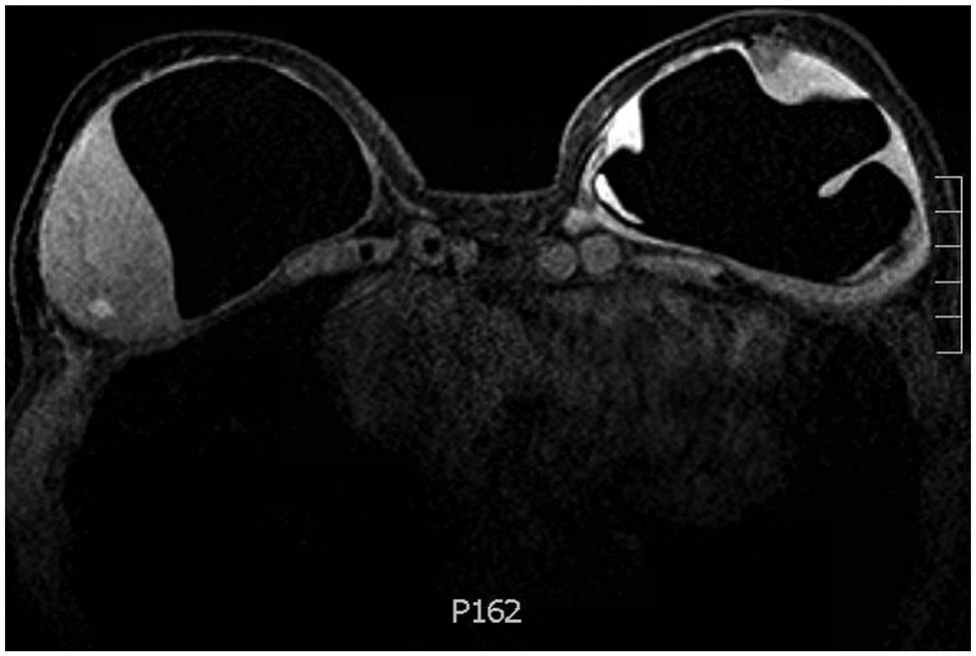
T1 weighted contrast bilateral breast MRI demonstrating a soft tissue intensity mass-like area attached to the right breast capsule with internal enhancing foci and trace periprosthetic fluid, in addition to enhancing foci at the deep margin of the left breast capsule.
